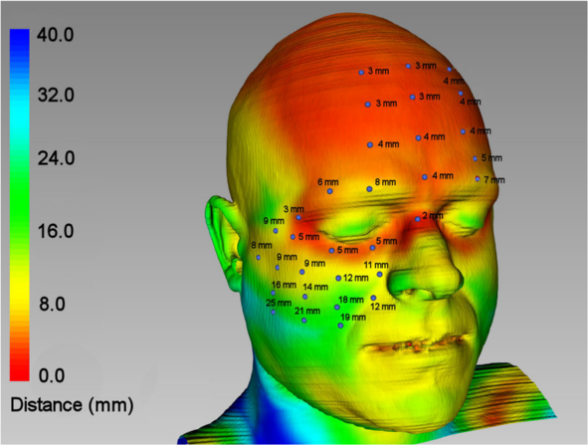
Soft tissue thickness map based on CT measurements. All values are rounded. Values lie between 3 to 4 mm in the forehead, whereas up to 25 mm are reached in the cheek region
